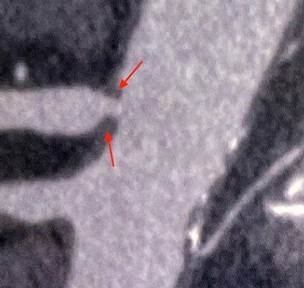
Computed tomography angiography demonstrates severe stenosis at the origin of the celiac trunk (red arrows), characterized by tapered contrast opacification.
